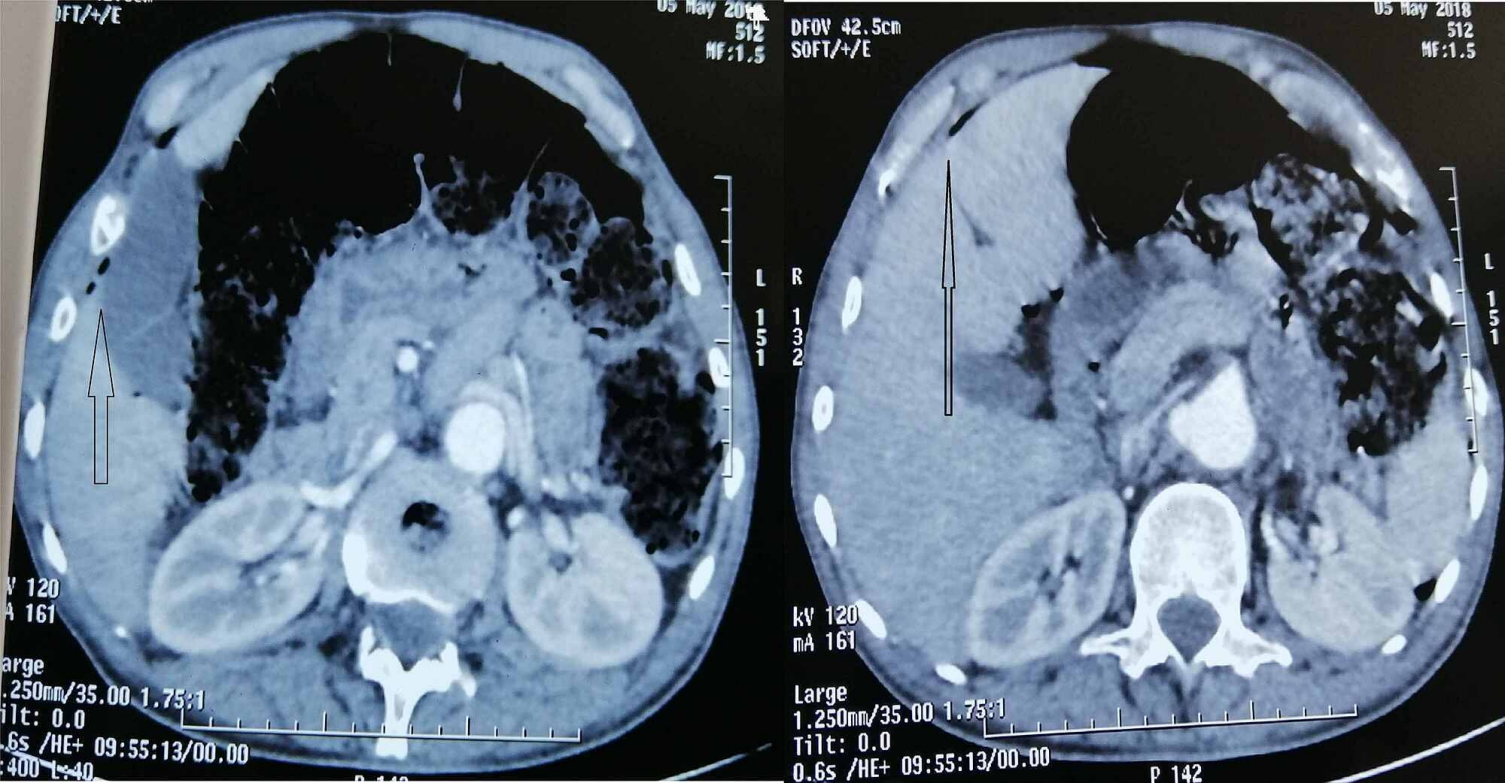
Abdominal CT scan Axial CT scan of the patient on admission showing free air in the peritoneal cavity.
